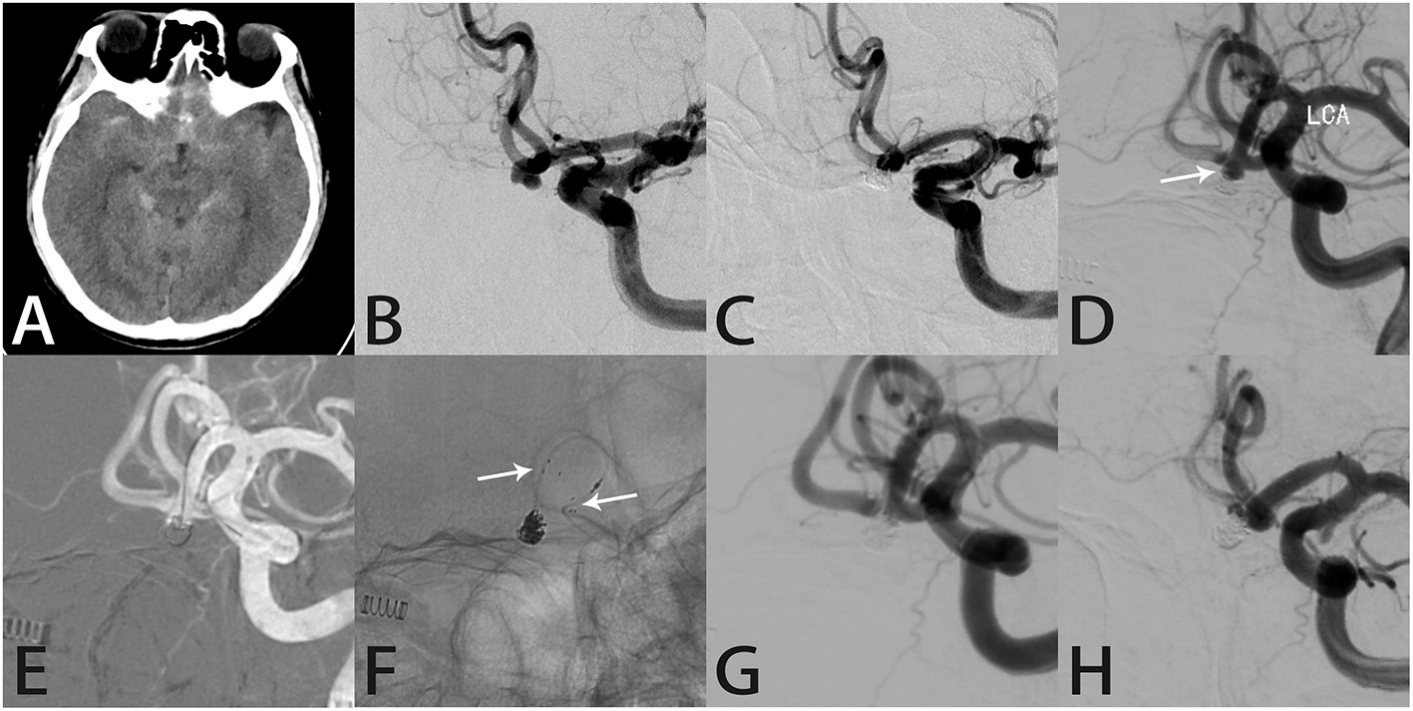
Images from a 57-year-old man with an anterior communicating artery aneurysm (case 2). (A) Computed tomography showed subarachnoid hemorrhage in the longitudinal and right Sylvian fissures as well as around the brainstem. (B) Preoperative angiography showed an anterior communicating artery aneurysm. (C) The aneurysm was occluded completely after coil embolization. (D) Follow-up angiography 54 months later showed an obvious recurrence in the aneurysm neck (white arrow). (E) During re-treatment, an Echelon 10 microcatheter (Medtronic, Dublin, Ireland) was delivered into the aneurysm sac to place the coils. (F) Intraprocedural angiography showed the three radiopaque markers (white arrows) at the proximal and distal ends of the Atlas stent (3.0 × 15 mm) and the coils within the aneurysm sac. (G) Angiography immediately after the procedure showed the aneurysm was occluded completely. (H) Follow-up angiography 8 months later showed complete aneurysmal occlusion and parent artery patency.
